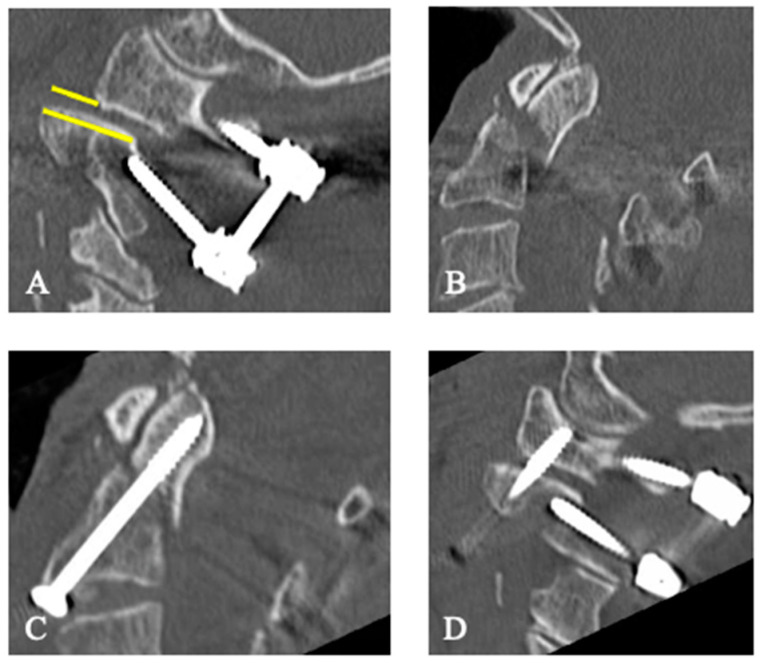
After dorsal surgical stabilization of Case 1 (Figure 1), there was a secondary dislocation with C2-uncovering ((A), yellow line) with dislocation of the odontoid (B). Ventral screw osteosynthesis of the odontoid (C) and additional fusion of C1 and C2 were performed (D). The postoperative CT scan shows the correct position in the C1/C2 joint (D).
